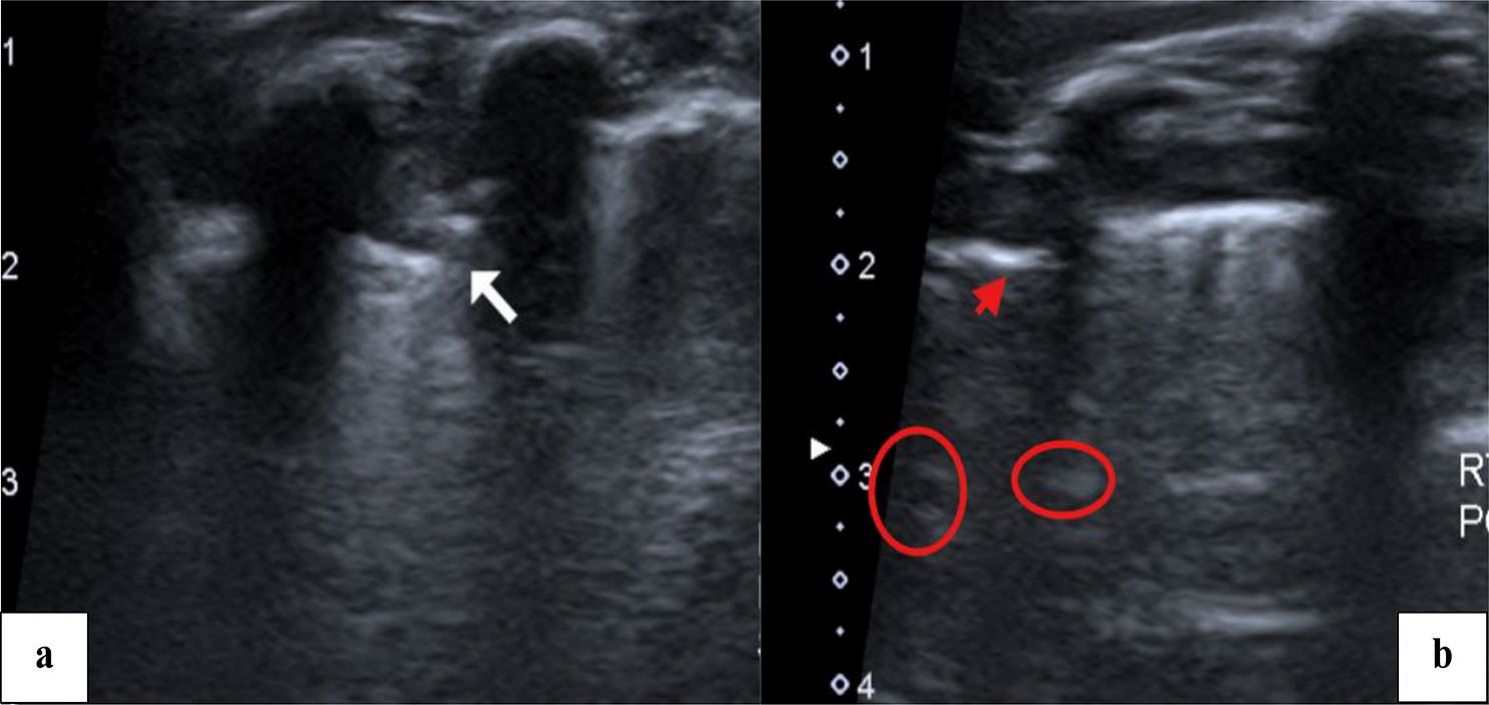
A 3-month-old female child with complicated community-acquired pneumonia. (a) Lung ultrasound examination done with the probe placed at the right lung, zone 1 showing loss of A-lines with consolidation of the lung, pleural thickening, and irregularity, arrow. (b) Lung ultrasound examination with the probe placed at zone 3 of the right lung showing transition point on the lung surface where the arrow represents absent lung sliding, denoting presence of pneumothorax. Fluid bronchogram is also noted (circles)
